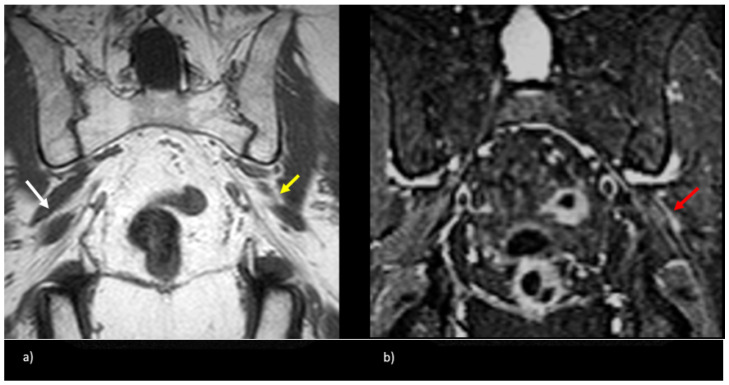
Patient presented with left-sided symptoms and lumbosacral plexus MRN showing bilateral type II variants: (a) coronal T1-weighted sequence showing right-sided sciatic nerve split around the normal right (white arrow) and atrophied left (yellow arrow) piriformis muscle; (b) coronal STIR sequence demonstrating increased signal of the left sciatic nerve (red arrow).
